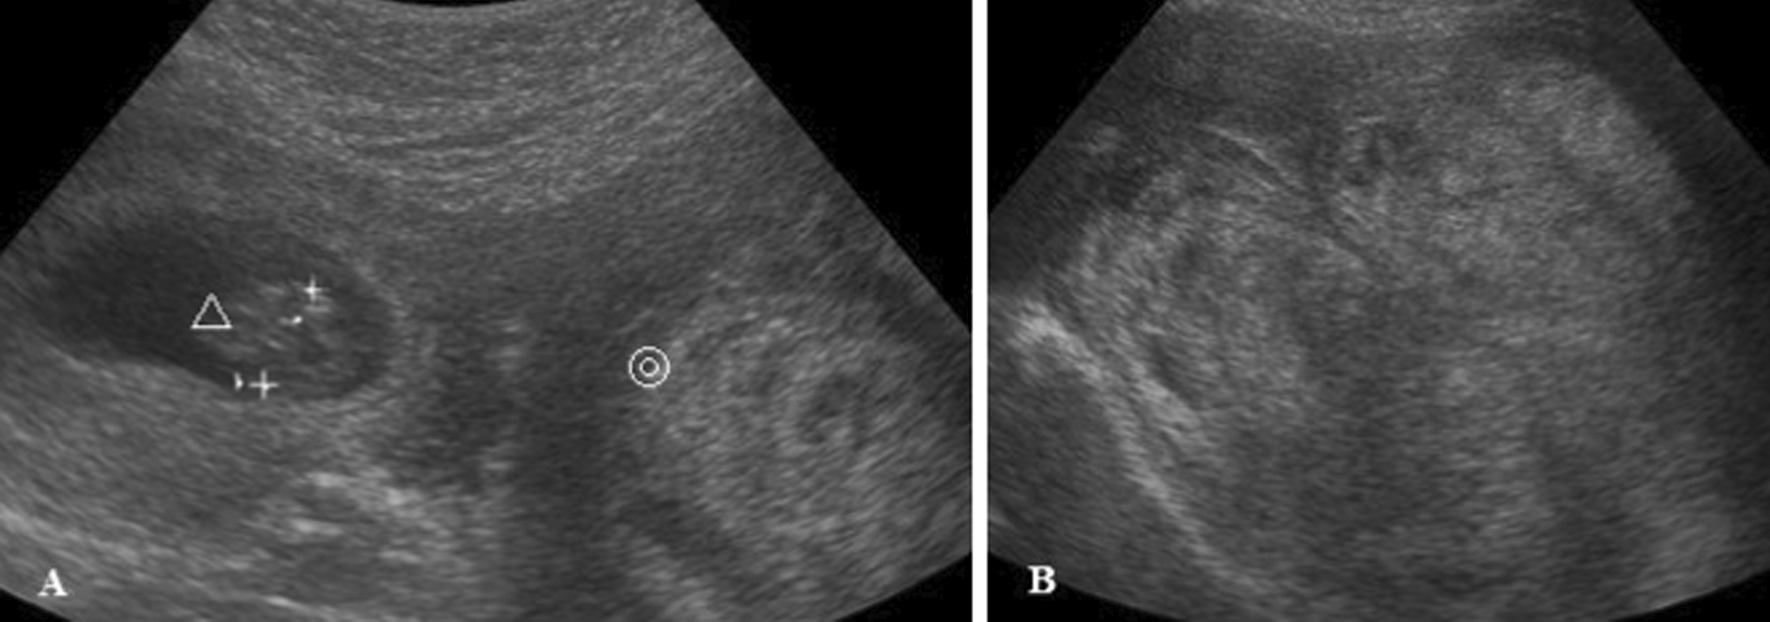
Transabdominal ultrasonography at 9 weeks of gestation. △ Fetus, ◎ Tumor. A The uterine body was shifted superiorly by the tumor. B Tumor size was 130 mm. It had a hyperechoic region, mainly with a partially low region
